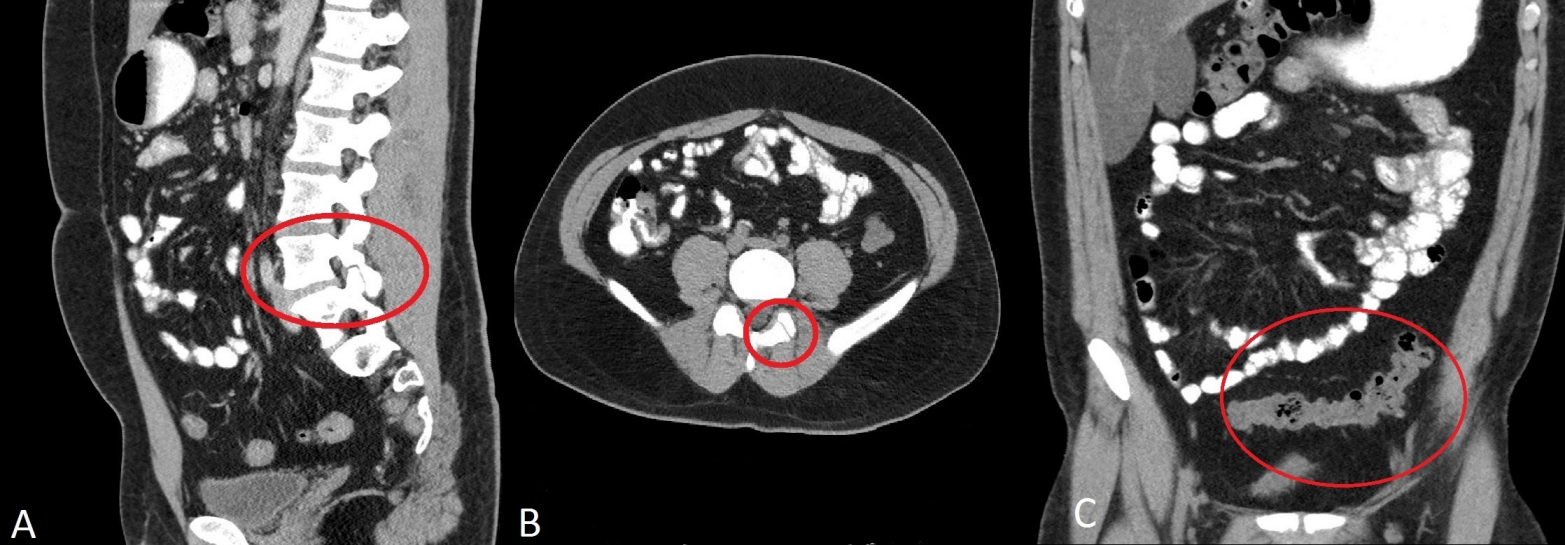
CT scan of abdomen and pelvis [A] Sagittal view [B] Axial view show unilateral pars interarticularis defect at the L4 level on the left side. [C] Coronal view demonstrates mild diverticulosis in the sigmoid colon without any evidence of diverticulitis.
